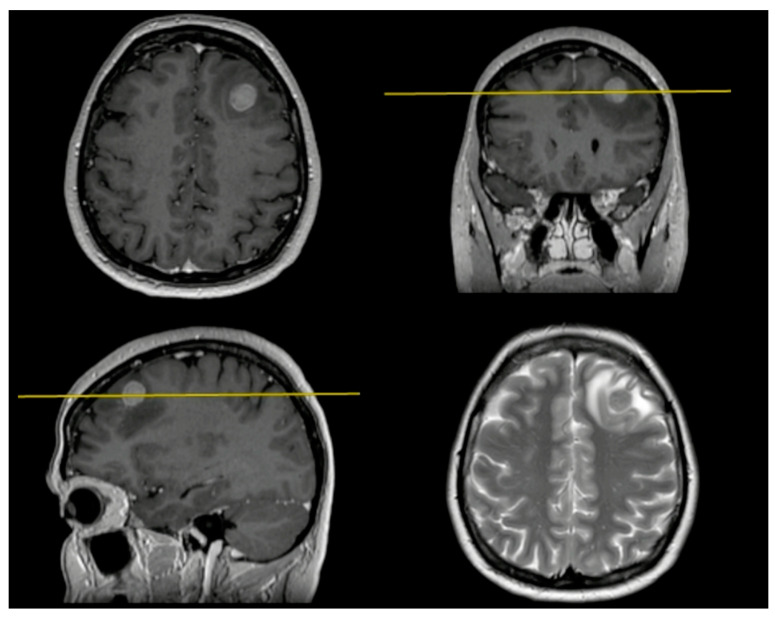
Preoperative MRI shows a BM located under the left middle frontal gyrus.
